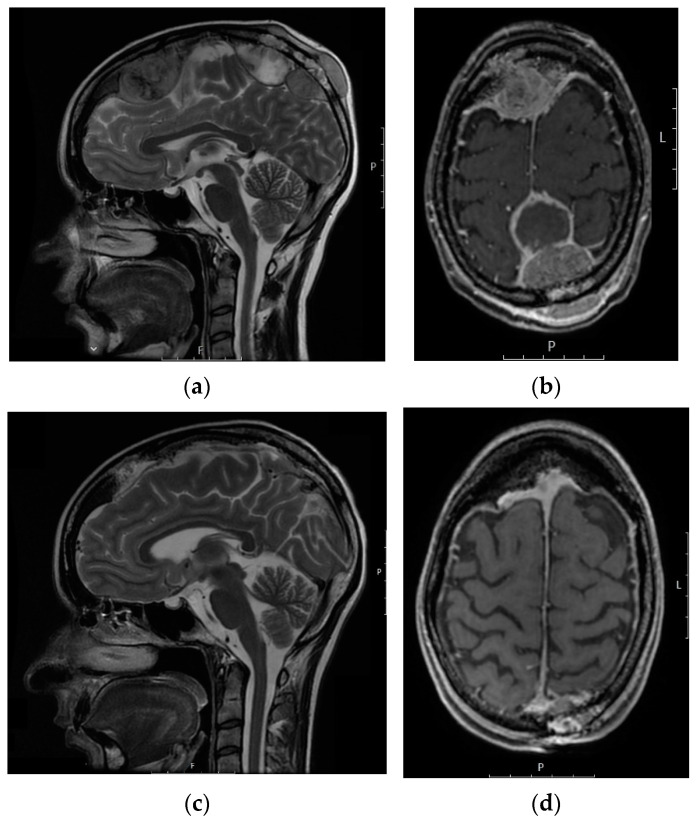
Axial T1-post IV contrast (a) and sagittal T2-weighted (b) MRI images of a 21-year old female with alveolar soft part sarcoma at the diagnosis of two large brain metastases with meningeal involvement and known skull bone metastases. The patient was treated with whole-brain radiotherapy (WBRT) and several different systemic therapies. Axial T1 (c) and sagittal T2-weighted (d) MRI images performed 2 years after WBRT, showing a very good response to treatments.
